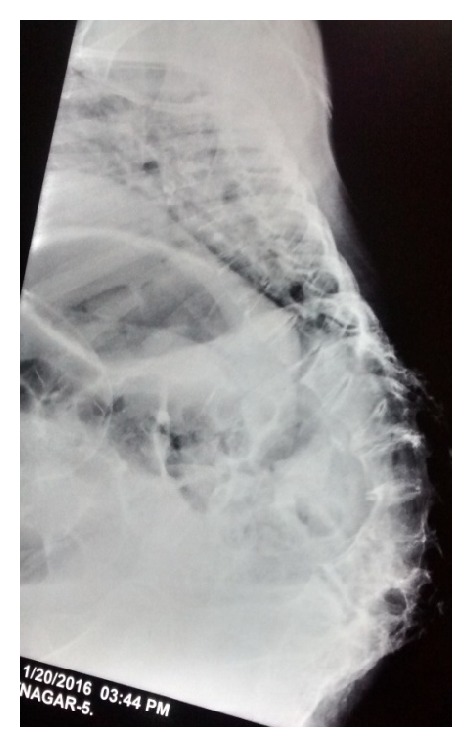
X-ray of spine showing kyphosis in lumbar region and lordosis in thoracic region.
